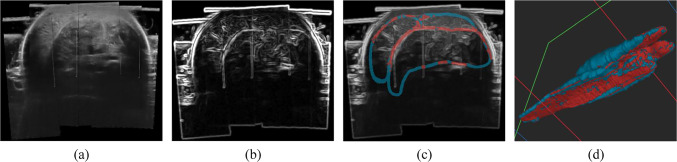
Distinct (red) and completed (blue) borders. a Ultrasound image, b Edges on the image, c 2D cross-sectional view with borders, and d 3D view of the borders
